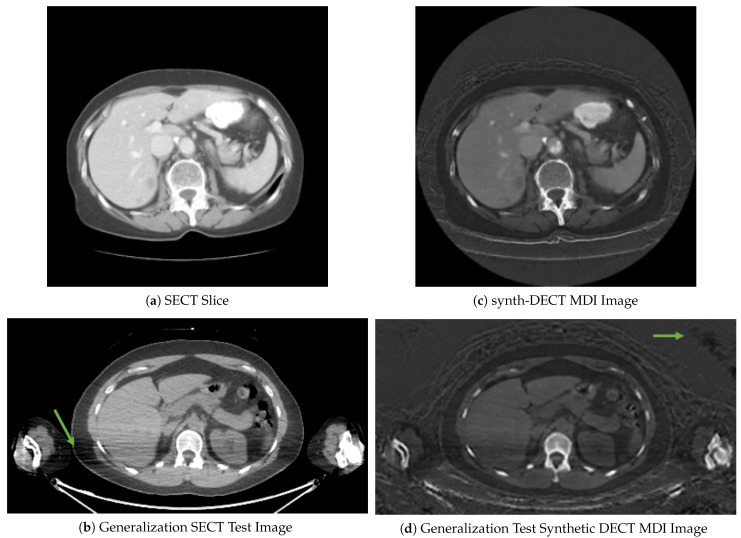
Cross sectional axial slices comparing the image-to-image translation for scans in CT-ORG. (a) A single axial slice from a patient single energy CT (SECT) scan. (b) Representative slice from one of the nine PET/CT scans used as the generalization test set: The streaks pointed to by the arrow are photon starvation artifacts that result from excess attenuation caused by the arms being at the side during the scan. (c) The synthetic dual energy CT material density iodine (synth-DECT MDI) image for the slice shown in (a). (d) The synth-DECT MDI image of the slice is shown in (b). The arrow in the synthetic slice shown in (d) points to a region in the air surrounding the patient that was distorted.
